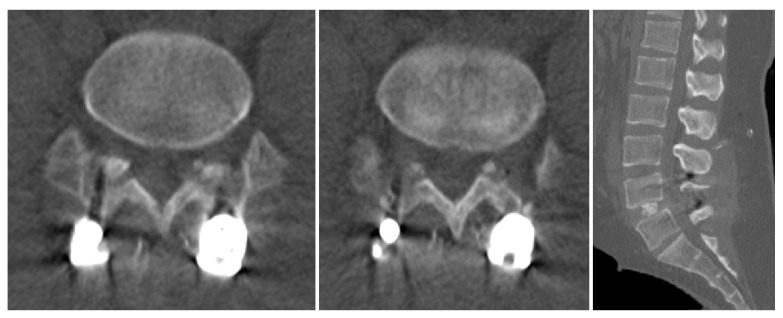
CT image after the aggressive decompression and fusion surgery at L4/L5.
